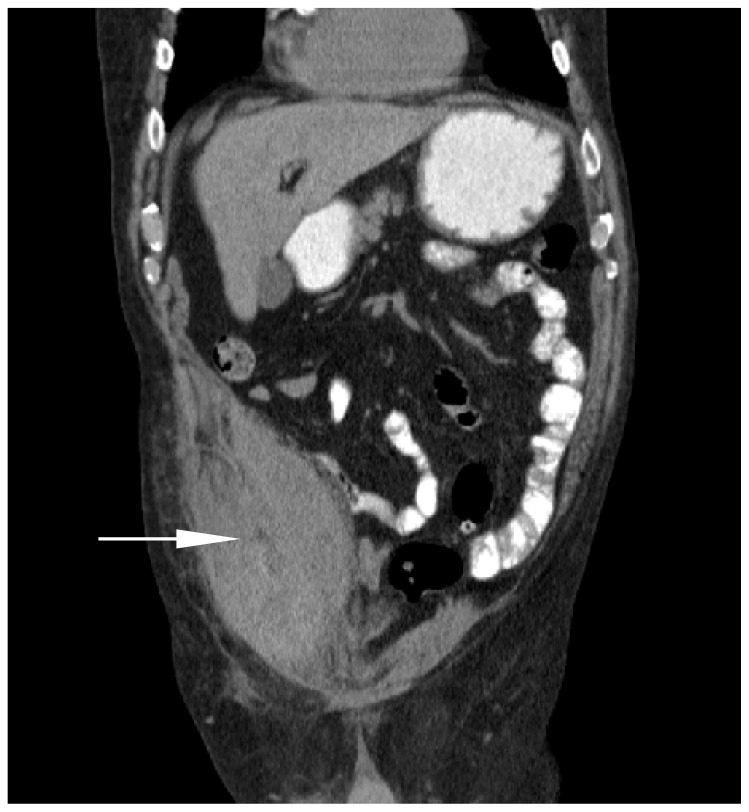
Coronal view computed tomographic scan revealing hematoma within the rectus sheath (arrow).
